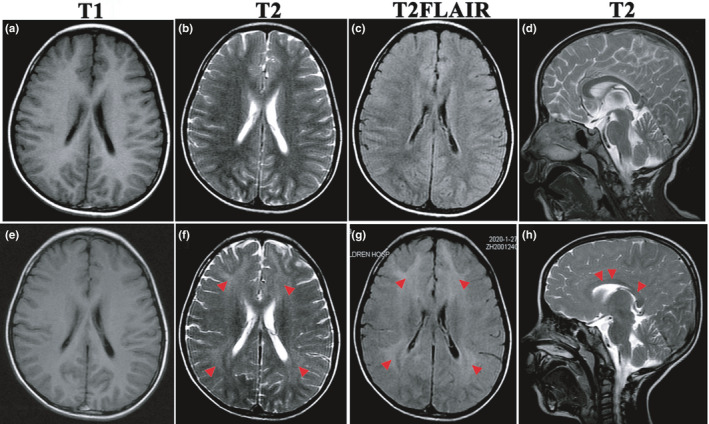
Dynamic presentation of brain MRI of Case 1. The MRI was normal at 1 month after the onset (a–d) and demonstrated bilateral and diffuse lesions in the cerebral white matter and corpus callosum (f–h, red arrowheads) 2 months later (e–h)
